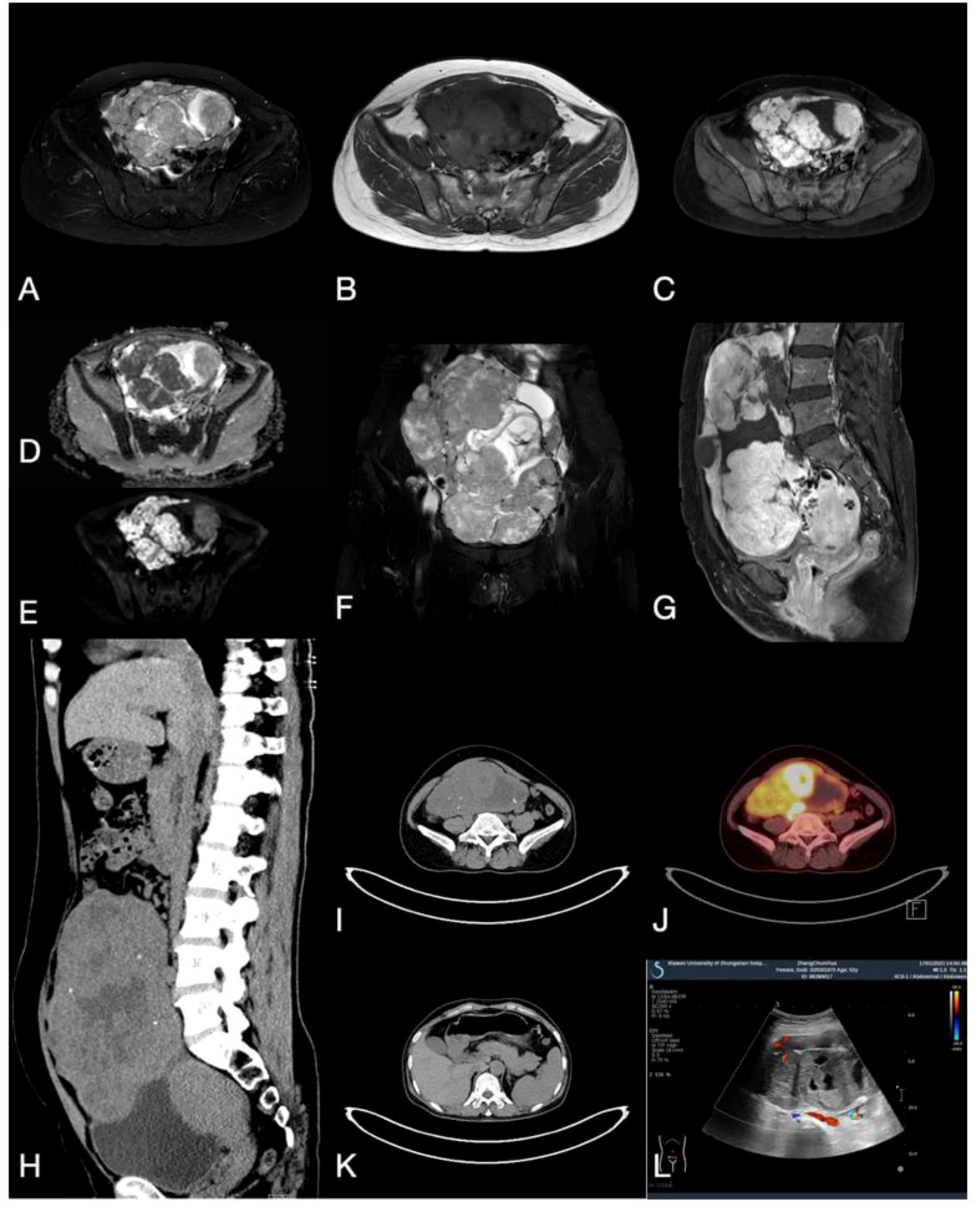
(A, B) MRI T2 and Tl weighted imaging revealed a cystic solid mass with mixed signals within the ovary. (C) MRI enhanced transverse imaging. (D, E) Diffusion-weighted MRI, ADC map. (F) Coronal T2 weighted imaging. (G) MRI enhanced sagittal imaging. (H, I) CT scan demonstrates a cystic-solid mass with mixed density and scattered calcification in the sagittal and transverse planes. (J) PET-CT indicates high uptake in the solid tumor component. (K) CT plain scan revealed no lesions in the pancreas, liver, or other regions. (L) Ultrasound indicates the presence of a cystic and solid mass with mixed echoes within the ovary.
